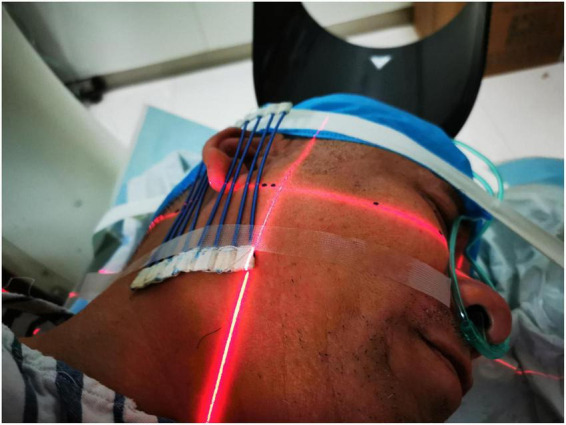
Placement of the CT positioning grids. CT positioning grids were placed anterior and posterior to the patient’s ear.
